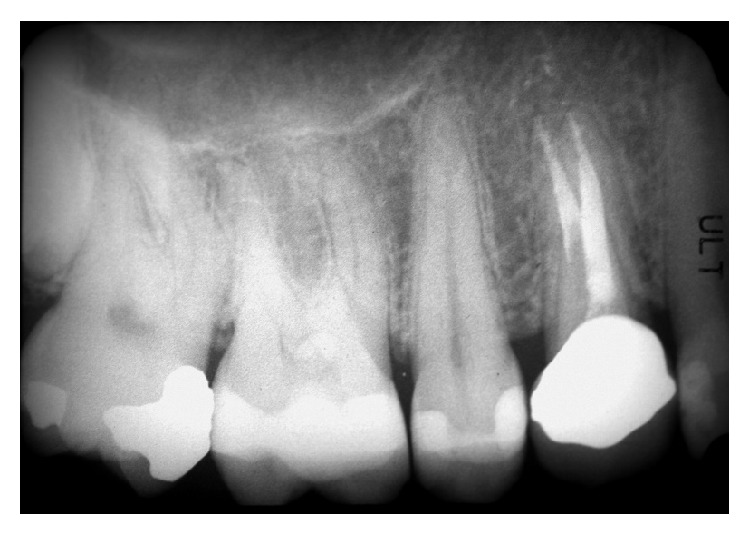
Periapical radiograph showed a fracture of the root, with a mesial well-defined lesion of the hard tissue of the upper right first premolar, as the radiolucent area affected the root surface of the tooth.
